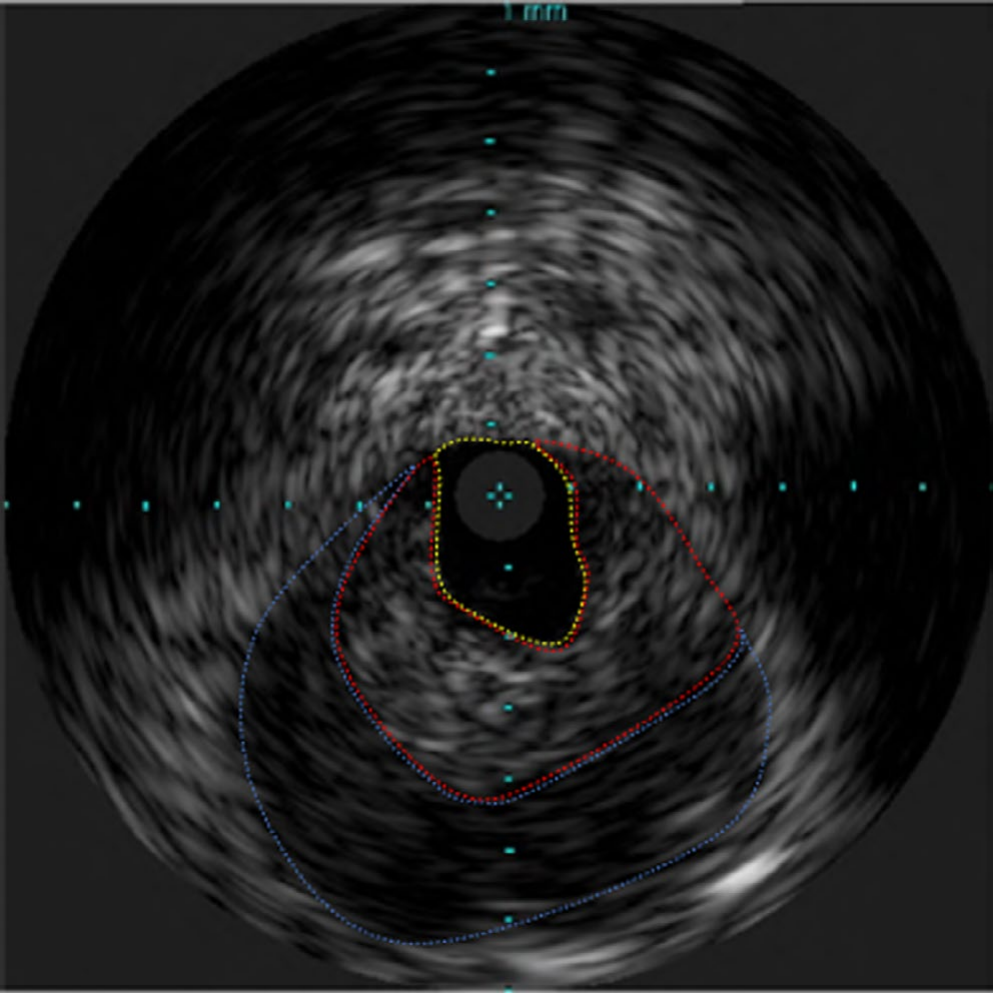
Intravascular ultrasound showed the true lumen was pressed upon by intramural hematoma. Yellow dotted line: true lumen. Red dotted line: hematoma. Blue dotted line: adventitia
